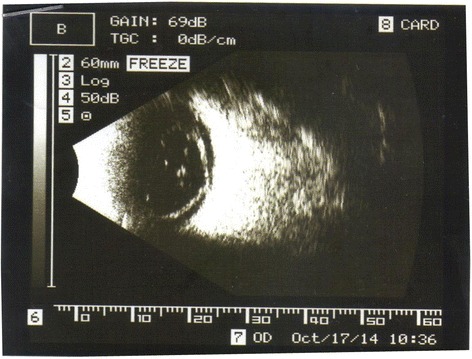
An ultrasound examination showed total retinal detachment
